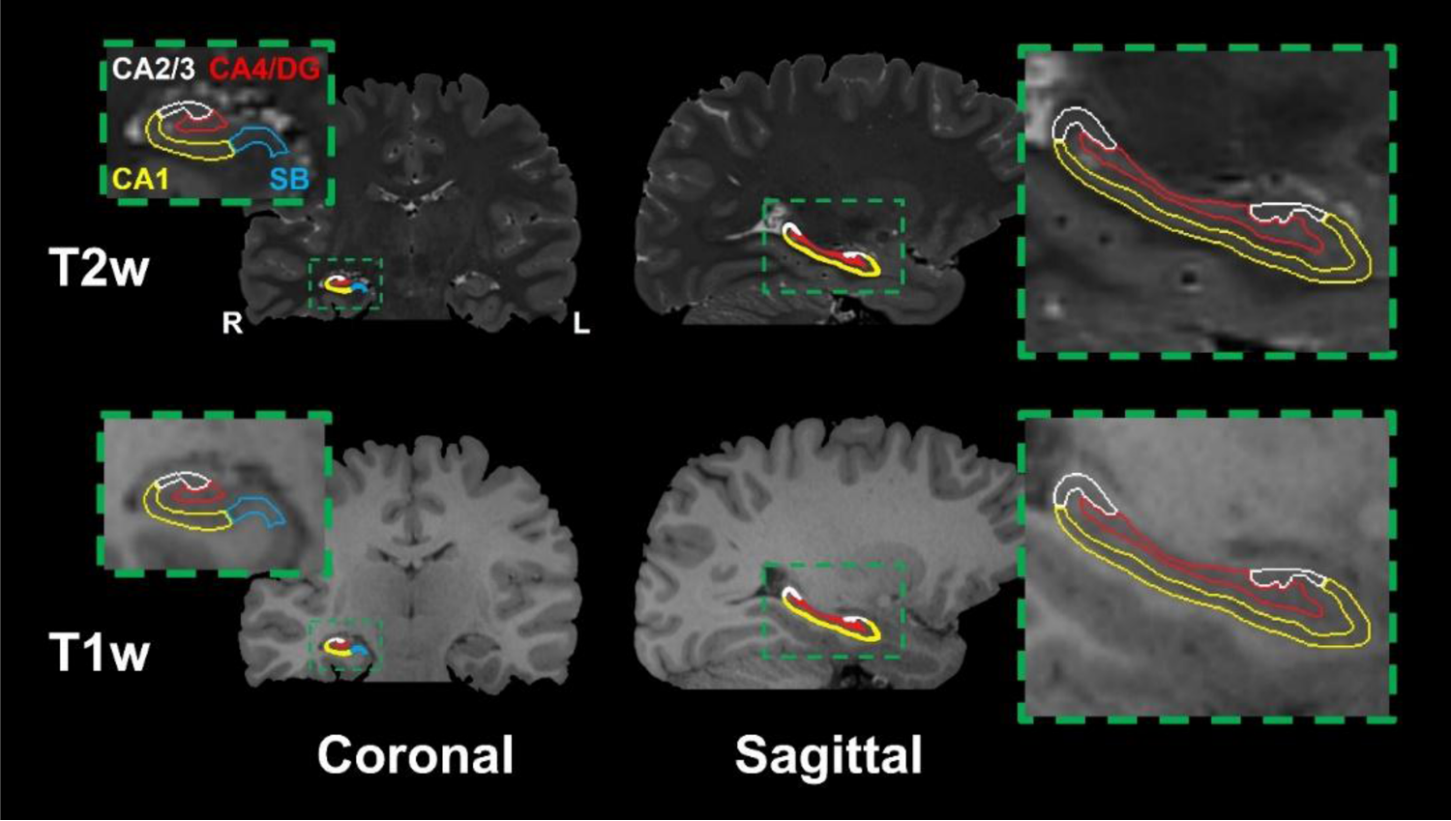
Coronal and sagittal T2-weighted (top) and T1-weighted (bottom) MR images from a healthy volunteer with insets highlighting the four hippocampal subregions used in this study: the subiculum (SB), cornu ammonis 1 (CA1), cornu ammonis 2 and 3 (CA2/3), and cornu ammonis 4 plus dentate gyrus (CA4/DG). Note that only the right hippocampus is labeled although both hippocampi were analyzed.
